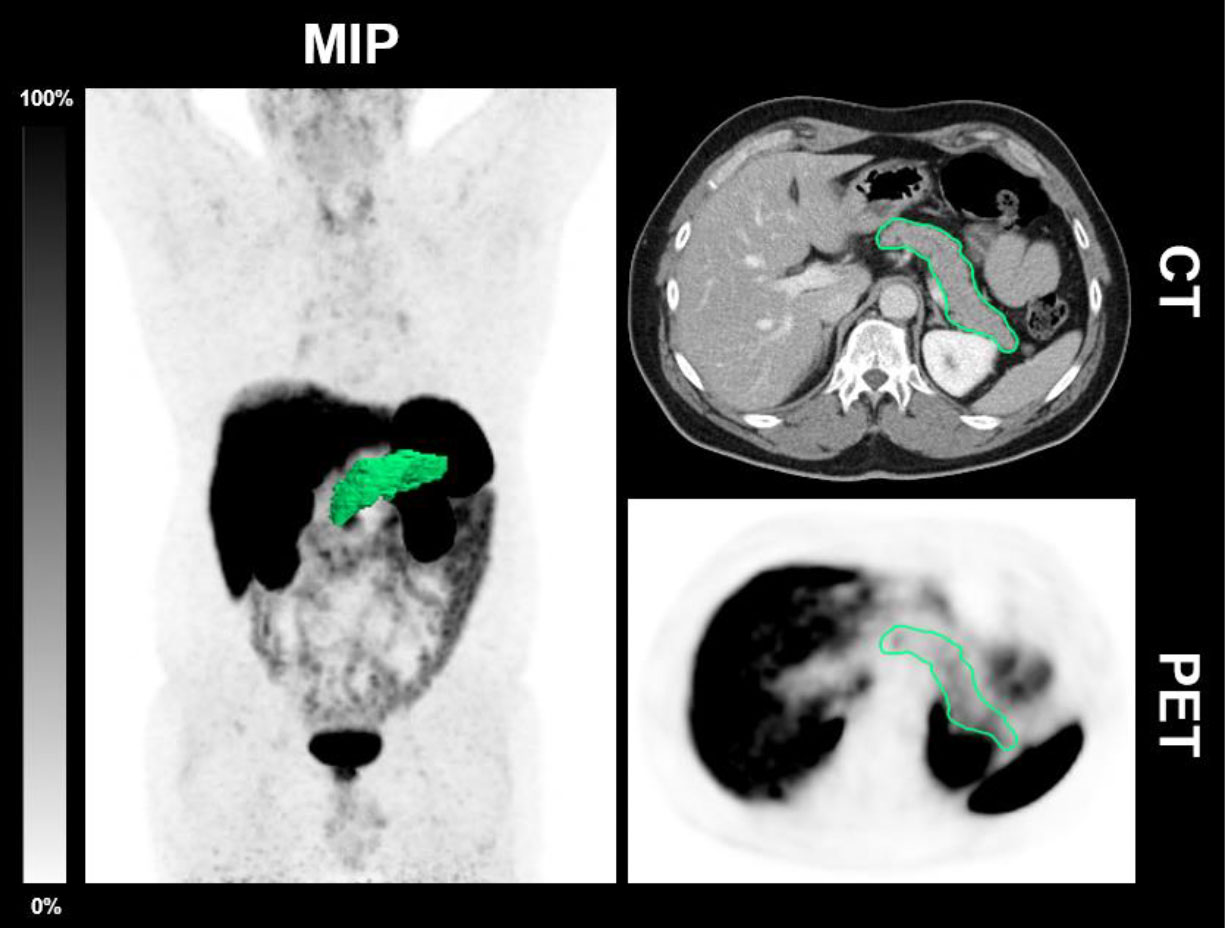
CT-derived delineation of the pancreas in a patient with T2D. Maximum intensity projection (MIP), axial CT and PET of a 56-year-old male patient diagnosed with T2D and antidiabetic medication with basal insulin supported oral therapy (Metformin and Insulin glargin) undergoing [^68^Ga]Ga-DOTATOC-PET/CT demonstrating the CT morphological delineation of the pancreas. HbA_1c_ was 6.9% (52 mmol/l); the SUV_max_ was 6.7 and the SUV_mean_ 2.4.
